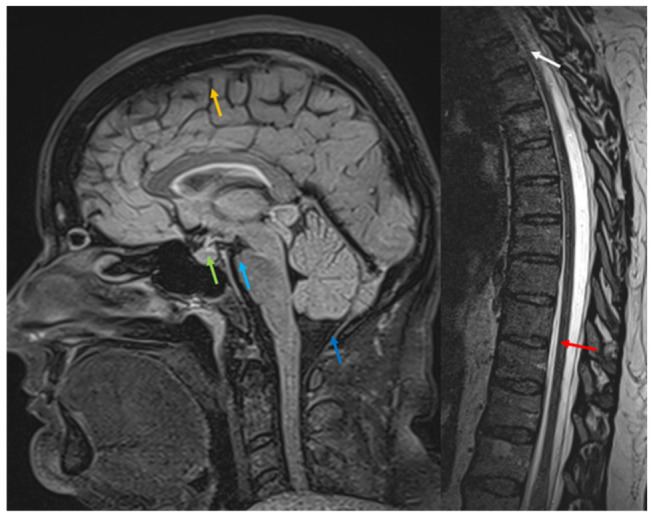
Six-month follow-up MRI after the procedure. Sagittal 3D FLAIR and sagittal T2 SPACE (0.8 mm) images are shown. The yellow arrow indicates that no further effusion or dural thickening is visible on follow-up imaging. The green arrow demonstrates resolution of previous pituitary gland enlargement, with improved visualization of the infundibulum and normal appearance of the pontomammillary distance (light blue arrow). The dark blue arrow shows no longer visible narrowing of the craniocervical junction, with no radiological signs of intracranial hypertension. On the T2 SPACE sagittal image, the previously noted thin dural double contour at the T8–T9 level (red arrow) demonstrates a reduction in the inter-dural content on follow-up imaging. At the cervicothoracic junction (C7–T1, white arrow), the dural duplication is no longer visible.
